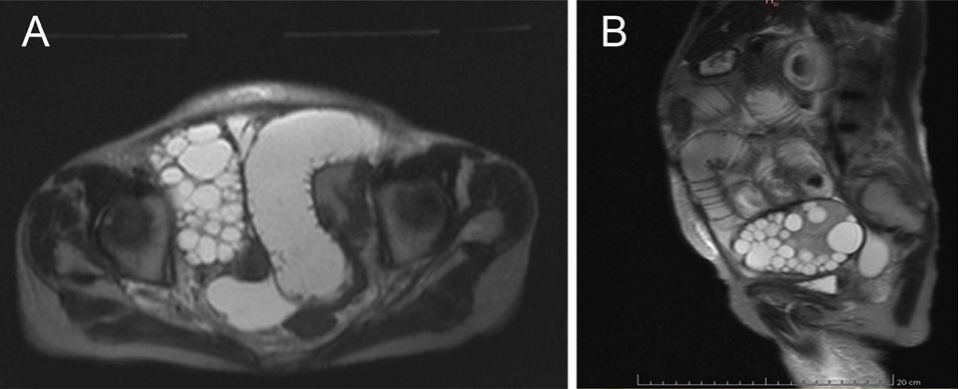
Axial (A) and sagittal (B) T2-weighted magnetic resonance image showing cystic mass with daughter cysts in the right ovary.
